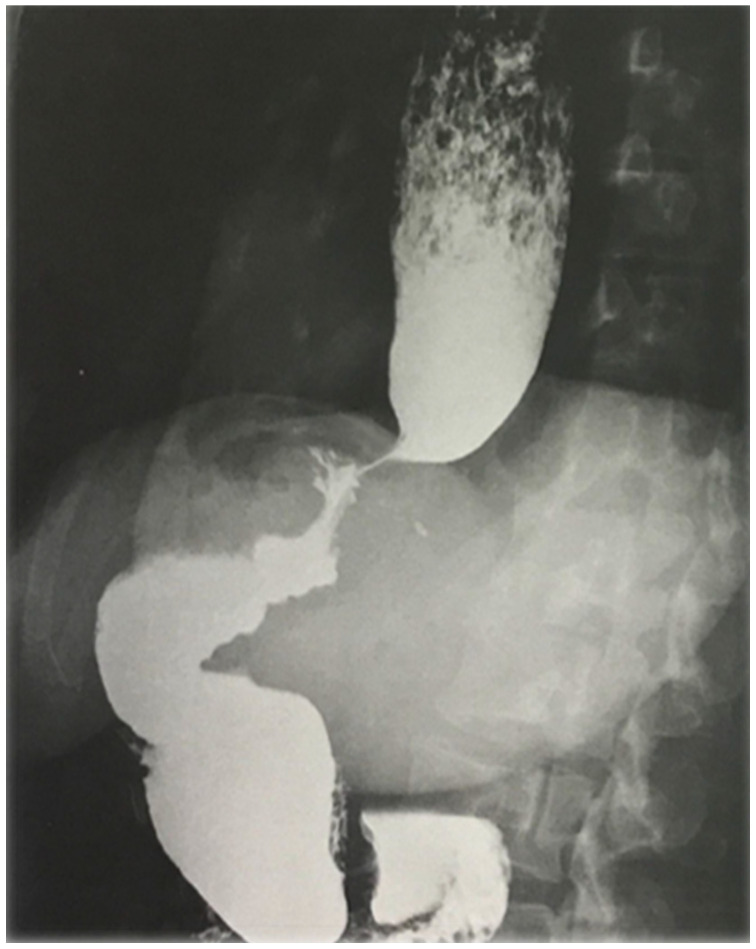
Barium esophageal X-ray showing marked esophageal dilation and contrast stasis suggestive of achalasia. Gastric sleeve appears regular.
